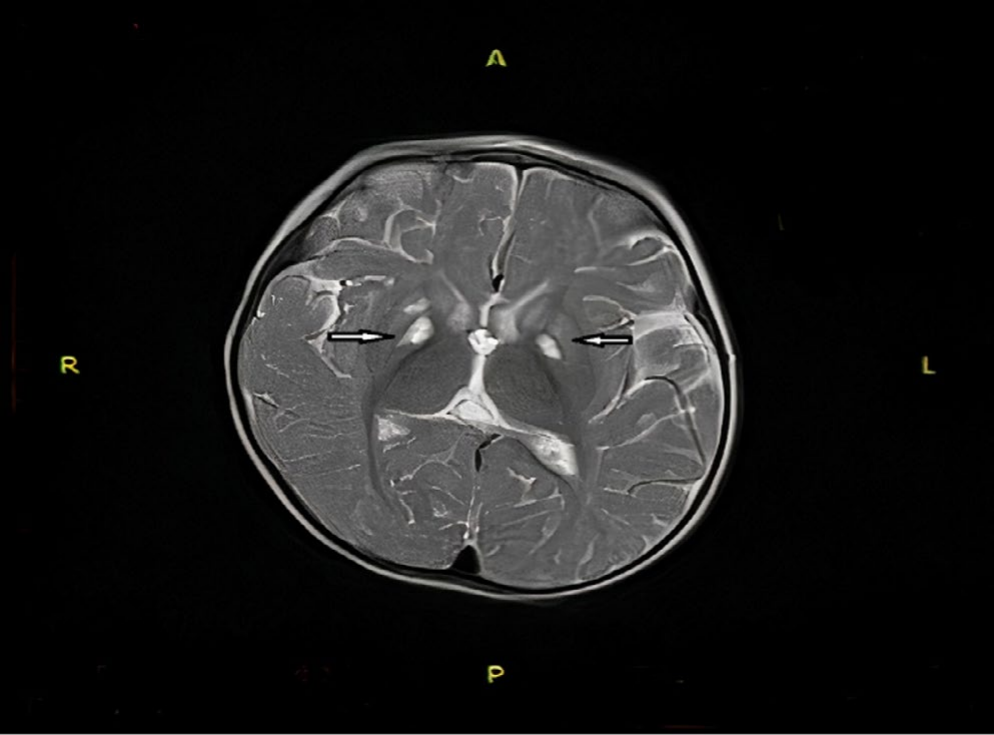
Brain MRI showed symmetrical hypersignal abnormality in the basal ganglia (white arrows)
